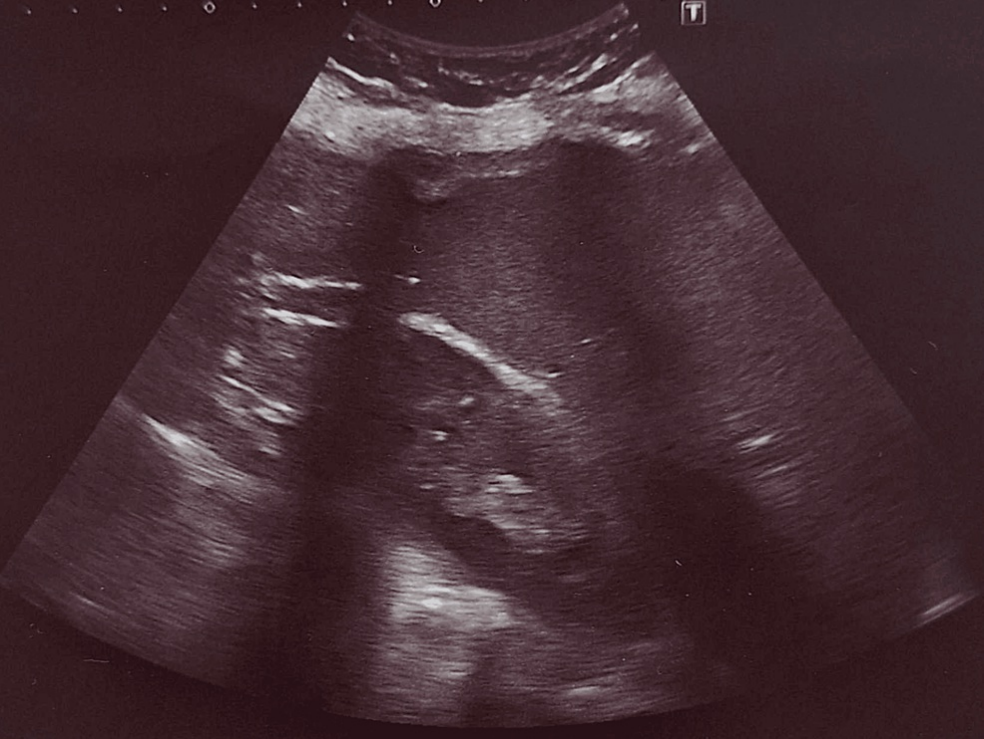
Postoperative ultrasound
